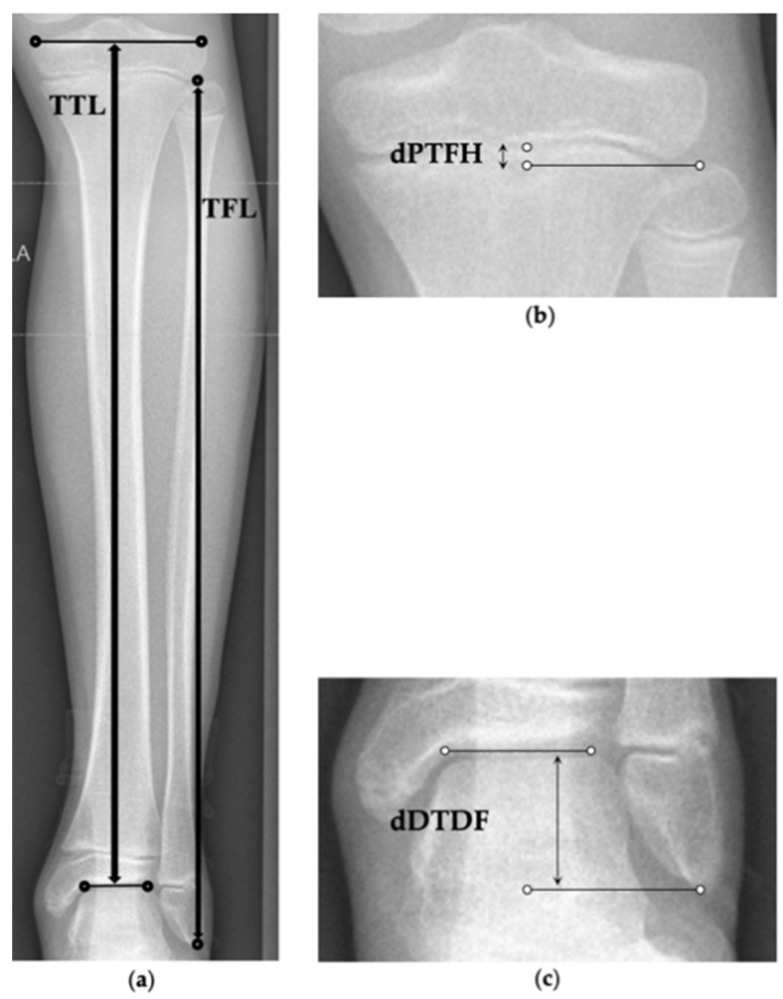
Radiographic measurement parameters. (a) TTL = Total tibial length measured from the joint line of the proximal to distal tibia (cm). TFL = Total fibular length measured from the tip of the proximal to the distal fibula (cm). (b) dPTFH = Distance between the center of the proximal tibial growth plate and a line tangential to the tip of the fibular head horizontal to the imaging plane (mm) [16]. (c) dDTDF = Distance between the articular surface of the distal tibia and a line horizontal to the imaging plane tangential to the distal tip of the fibula (cm) [18].
